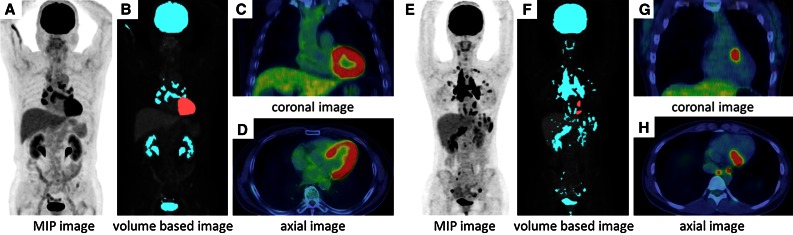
Representative cases. Figure (A-D) shows a 73-year-old man with diffuse LV FDG uptake, who was instructed to fast for a minimum of 6 h and whose FPG and FFA levels were 95 mg/dL and 464 μEq/L at baseline. Cardiac metabolic volume was estimated as 166.4 mL. There are multiple abnormal uptakes in mediastinal and hilar lymph nodes. Figure (E-H) shows a 25-year-old woman without diffuse LV FDG uptake, who had over 18 h’ fasting with a low-carbohydrate diet. Her FPG and FFA levels were 76 mg/dL and 1924 μEq/L at baseline. FDG PET/CT shows focal basal anterior wall uptake. Cardiac metabolic volume was estimated to be 8.3 mL. There are multiple lung uptakes and multiple lymph node uptakes in supraclavicular, mediastinal, hilar, and abdominal regions. MIP = maximum intensity projection
